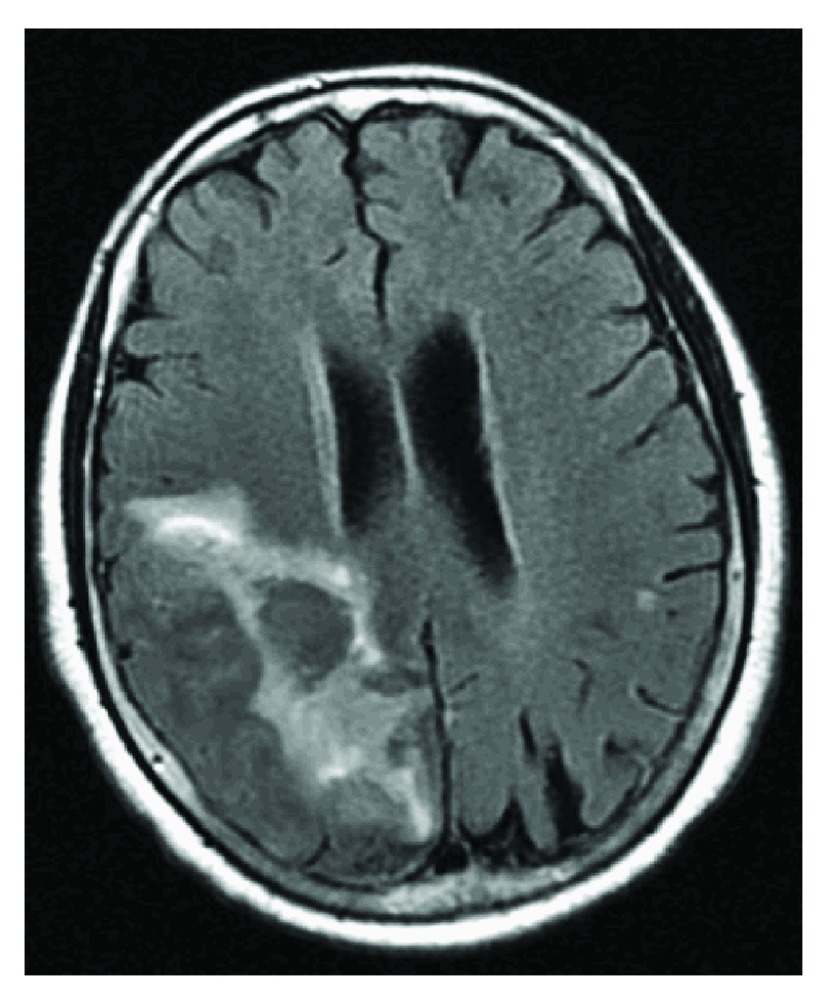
MRI-T2 Flair showing hyperintensity extending from posterior frontal, parietal causing mass effect compressing the midline and posterior corpus callosum.
